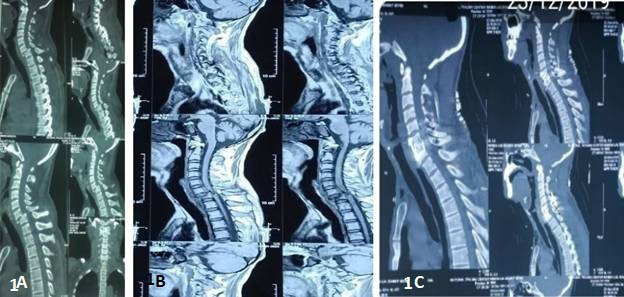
(A) Preoperative CT-Scan showing the fracture-dislocation of the C6-7 level. (B) Pre-operative MRI with significant anterior cord compression. (C) Postoperative CT-Scan showing adequate decompression, reduction, and fixation.
